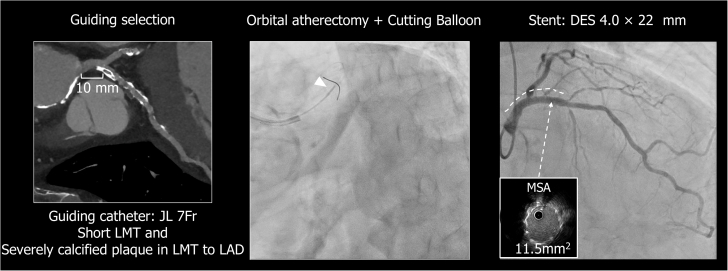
PCI Strategy and Procedural Outcome Images show CT-guided selection of the guiding catheter, the procedural steps of the PCI, and the final procedural result. CT = computed tomography; DES = drug-eluting stent; LAD = left anterior descending artery; LMT = left main trunk; MSA = minimum stent area; PCI = percutaneous coronary intervention.
